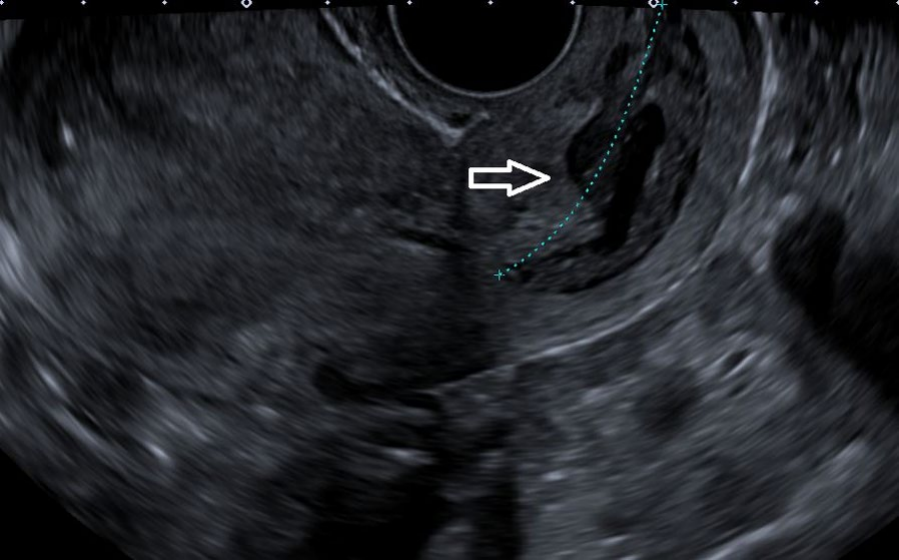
Transvaginal ultrasound showing an echogenic focus (shown by white arrow) adherent to right ovary with no vascularity
